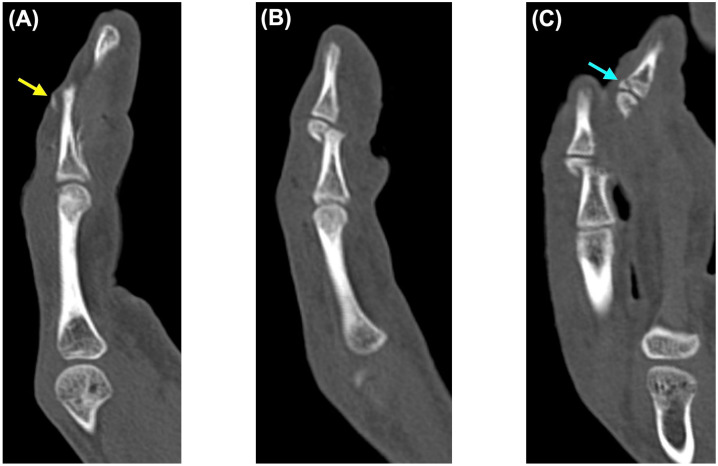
Preoperative computed tomography of the left ring and little fingers. (A) Sagittal image of the ring finger showing a tiny dorsal avulsion fragment at the base of the distal phalanx, consistent with bony mallet injury (yellow arrow). (B) Sagittal image of the little finger showing a middle phalanx fracture with a small distal fragment. (C) Coronal reconstructed image showing the spatial relationship between the ring and little finger injuries. The blue arrow indicates the bone fragment at the base of the distal phalanx of the ring finger.
